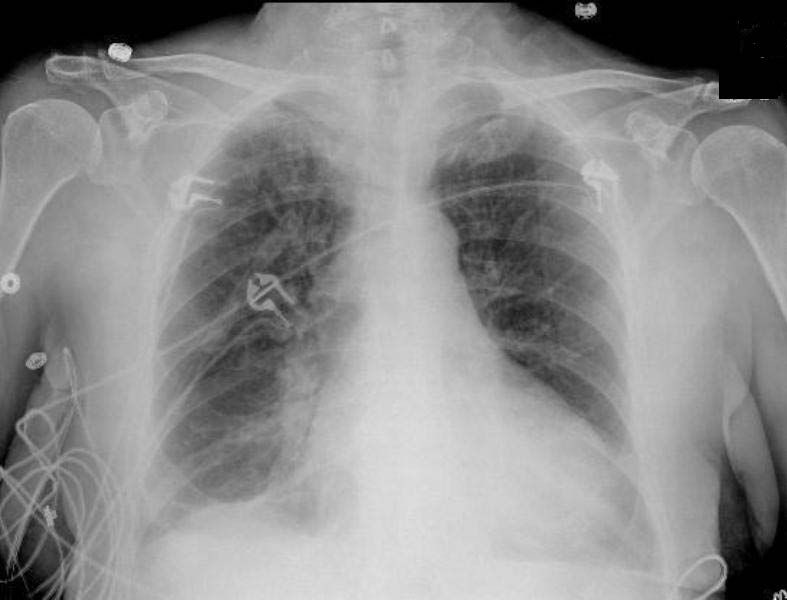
Chest X-ray showing bilateral pleural effusion and pulmonary venous congestion.
